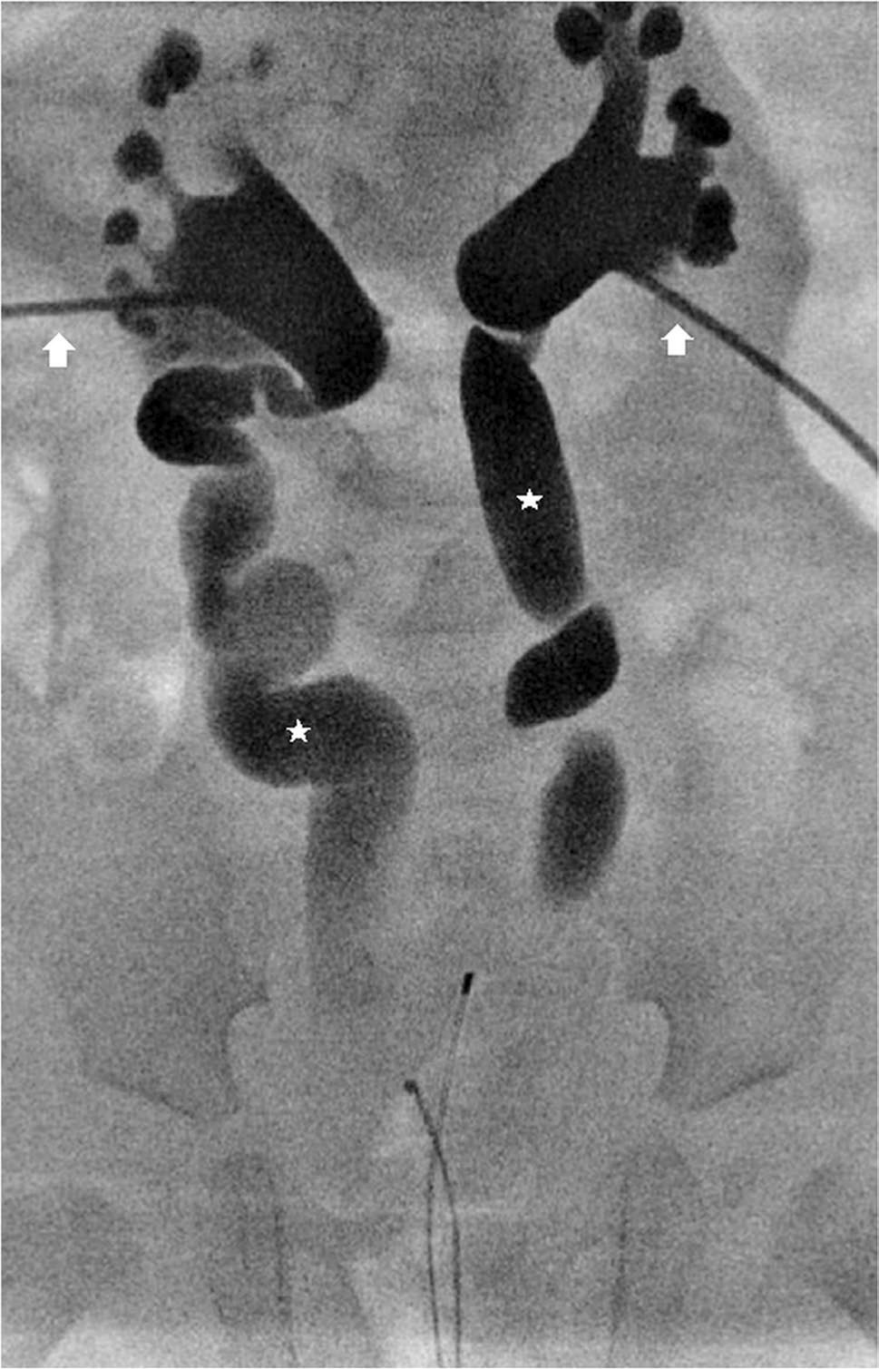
Double nephrostomy performed for bilateral stenosis post ureteral reimplantation in a male neonate. Anteroposterior radiograph with contrast agent administered through nephrostomy tubes (*arrows*) confirms the correct localisation of the catheters in the pelvicalyceal systems and shows dilatation of the urinary tract with enlarged ureters (*stars*). Case courtesy of Pr. Philippe Petit, Marseille, France
